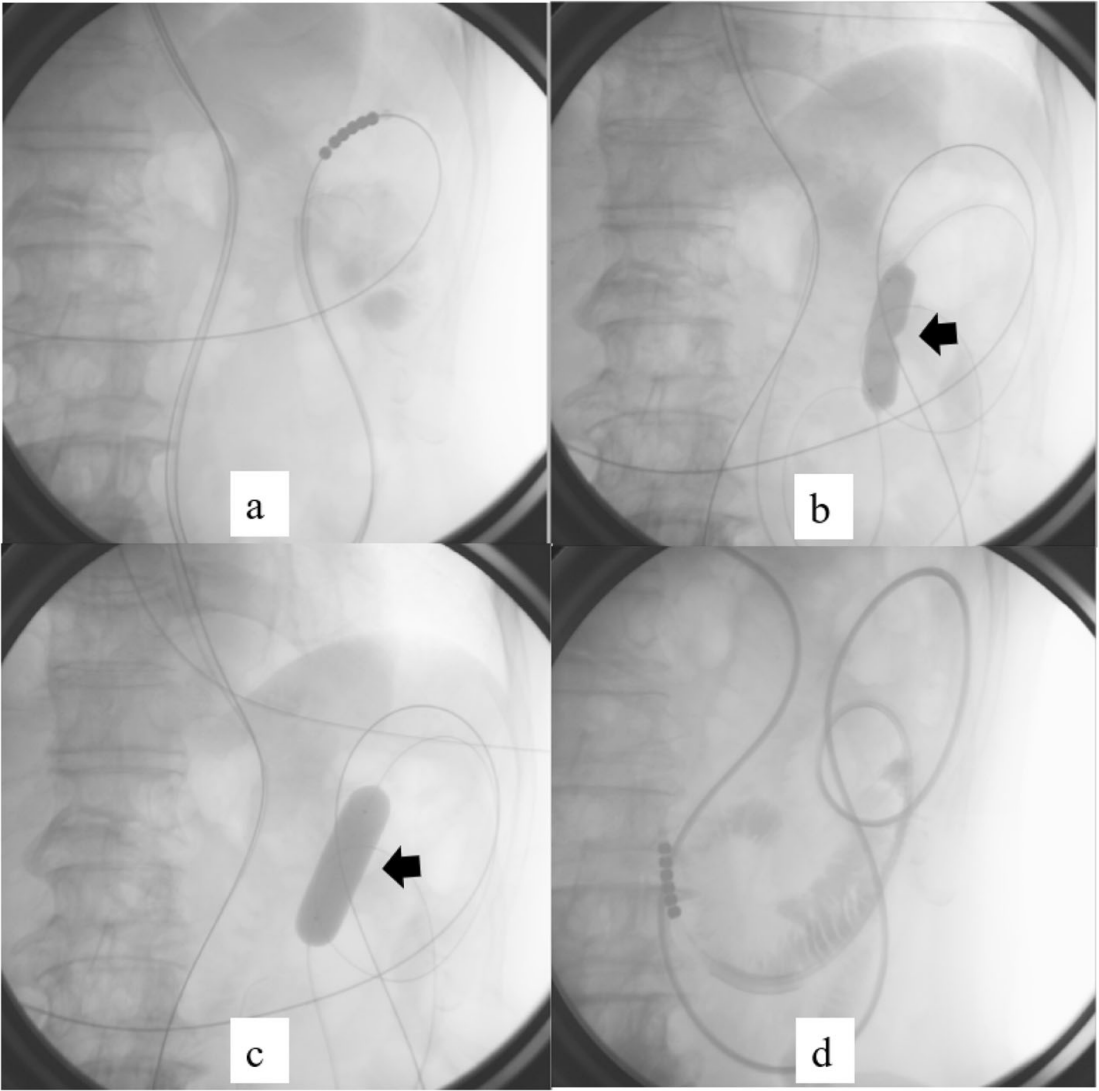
a, b, c, d Fluoroscopic balloon dilation procedure (imaging of case 3). a Radiograph showing insertion of the guidewire up to the site of stenosis. b Radiograph showing the “waist” caused by stenosis of the jejunojejunostomy (arrow). c Radiograph showing disappearance of the “waist” after balloon dilation (arrow). d Radiograph showing that the ileus tube could be passed across the site of stenosis
